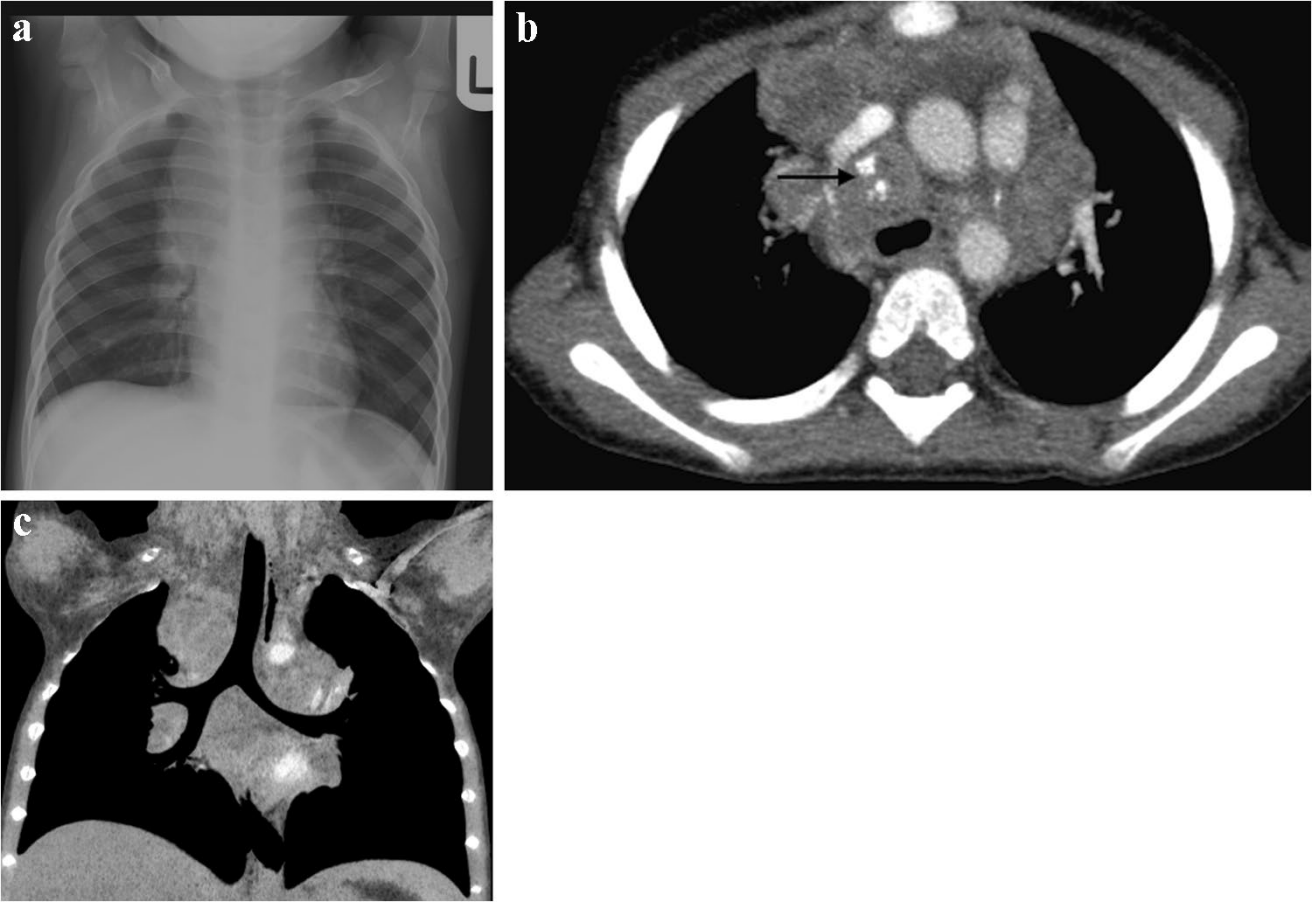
A 1-year-old girl with a cough and known to be a tuberculosis (TB) contact a Posteroanterior chest radiograph shows extensive mediastinal and hilar lymphadenopathy. b Axial post-contrast computed tomography (mediastinal window) performed a few days later confirms peripherally enhancing and low-density anterior mediastinal, pre-vascular, hilar and paratracheal nodes. There is also a pre-tracheal node that shows punctate calcification (*arrow*). These features are characteristic of TB. c Coronal thick slab multiplanar reconstruction (mediastinal window) demonstrates deviation of the trachea to the left but no significant airway compression
